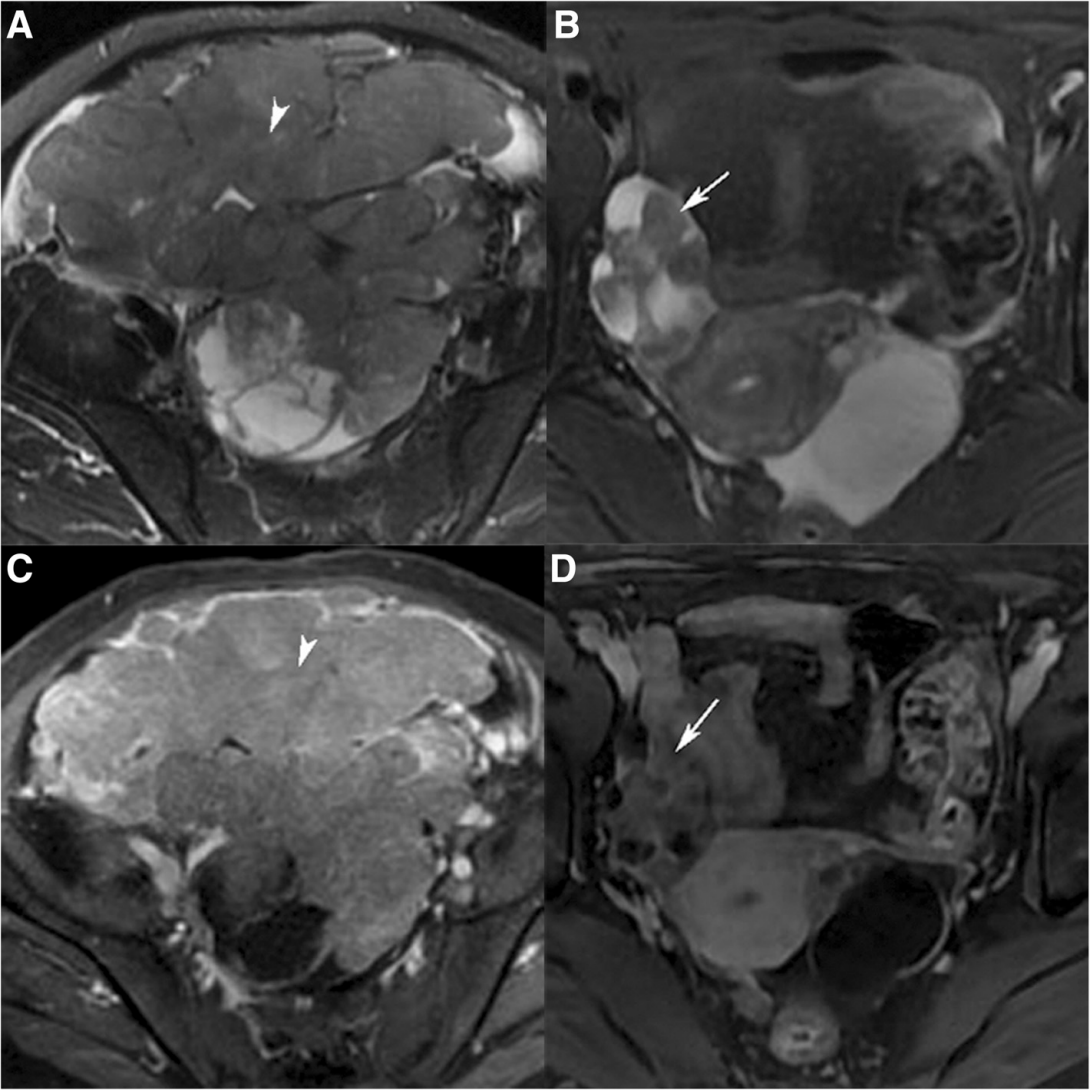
a and c A 58-year-old woman with serous cystadenocarcinoma on the right ovary. a Axial T2WI with fat saturation image showed the locules were not uniform. c Axial LAVA dynamic contrast-enhanced MRI image showed the solid portion was prominent enhancement (arrowhead). b and d: A 49-year-old woman with metastatic ovarian tumors on the right ovary. b Axial T2WI with fat saturation image showed the locules were uniform. d Axial LAVA dynamic contrast-enhanced MRI image showed the solid portion was moderate enhancement (arrow)
